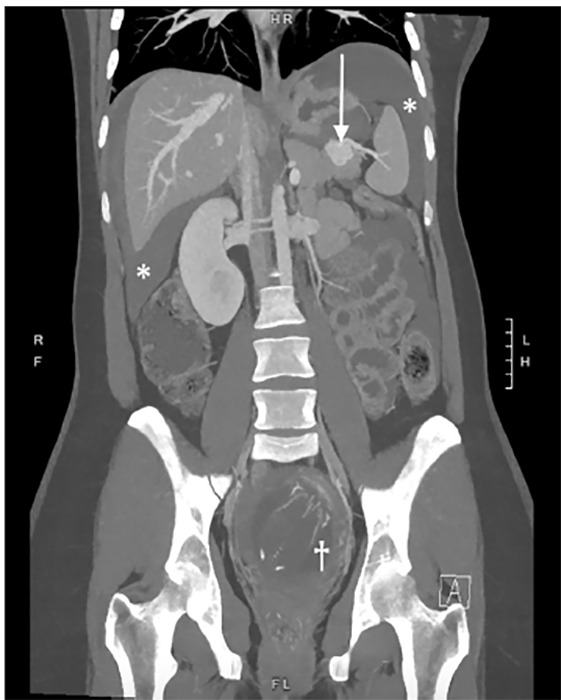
Computed tomography coronal reformat showing the ruptured splenic artery aneurysm (arrow) and significant hemoperitoneum around the liver and spleen (asterisks). The gravid uterus with developing fetus is also noted within the pelvis (dagger), a combination rarely seen on imaging due to the high mortality of this disease.
